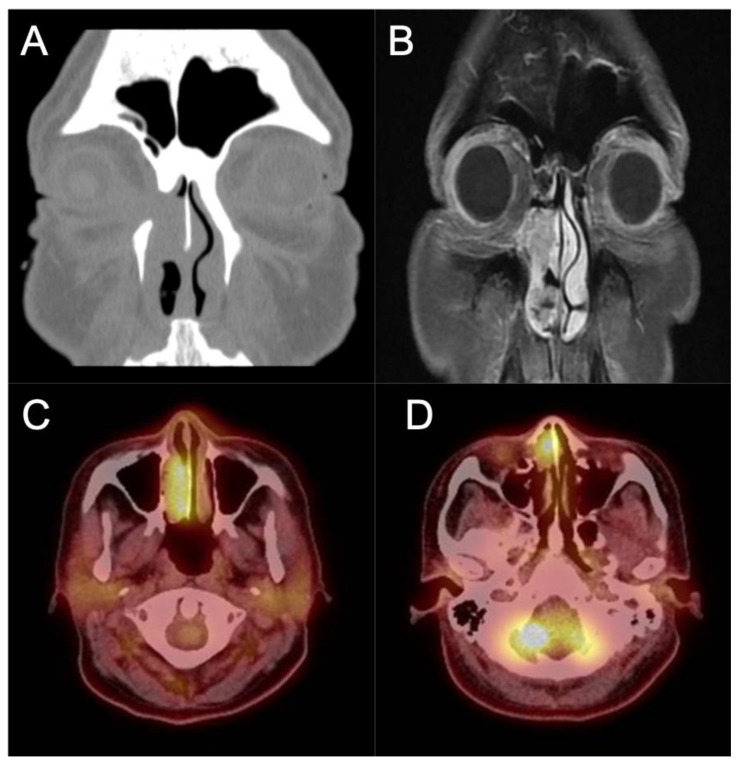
IP-degenerated squamous cell carcinoma. (A) Non-contrasted CT scan demonstrating an erosive mass in the right nasal cavity with invasion into the orbit. (B) T1 sequence contrasted MRI scan demonstrating the invasion of the mass into the medial orbit. (C,D) PET CT scan demonstrating avidity of the right nasal mass.
